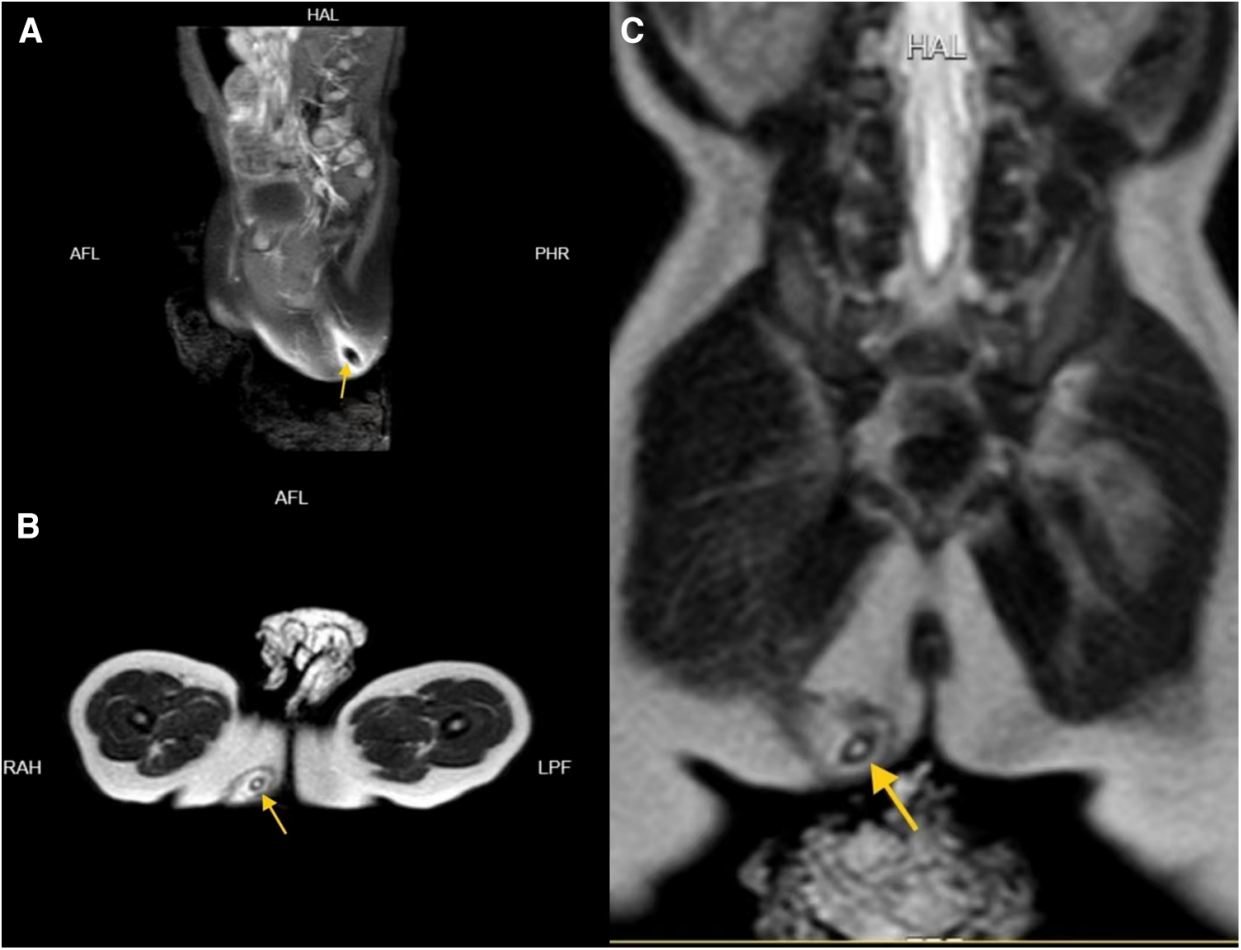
MRI images of patient 2 in the sagittal (A), horizontal (B), and coronal plane (C) showing the jujube pit (indicated by arrows).
